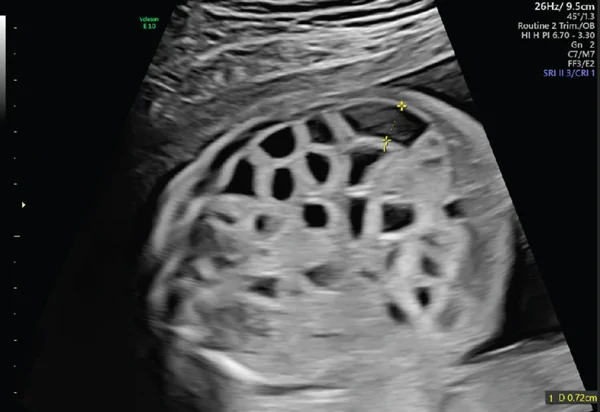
Case 1, dilated bowel loops at 23 weeks of gestation in patient with a SLC9A3 mutation (ultrasound image).
